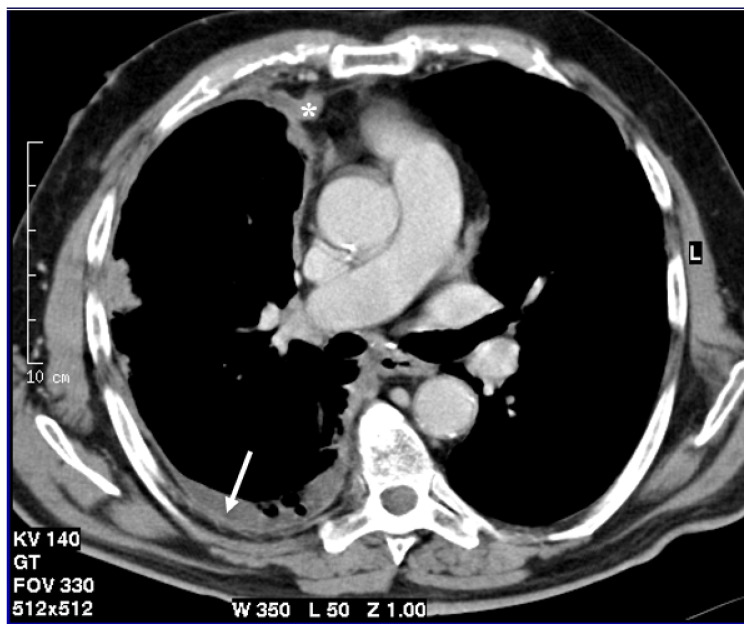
Axial CT scan after contrast administration reconstructed with smooth kernel with mediastinal windows demonstrates circumferential pleural nodular thickening with mediastinal pleura involvement (asterisk) and pleural effusion (arrow).
